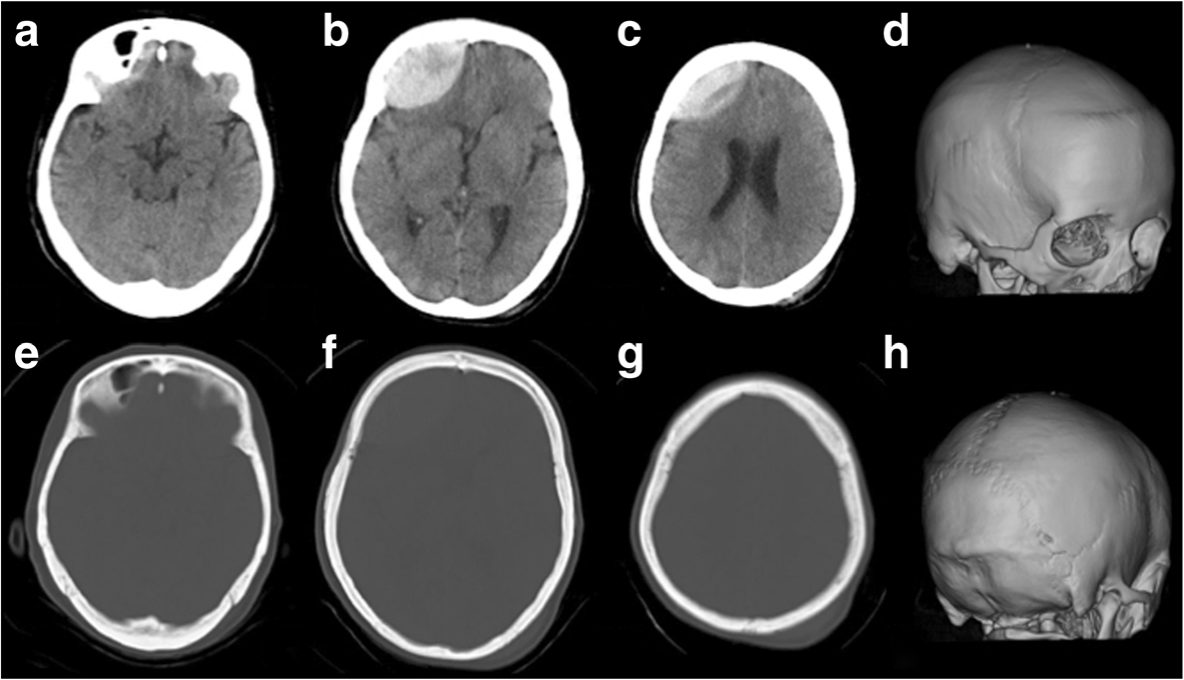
Head computed tomography (case 1). Computed tomography at 150 min after the injury showed acute epidural hematoma in the right frontal region (a-c) and no evidence of bone fracture (d-h)
